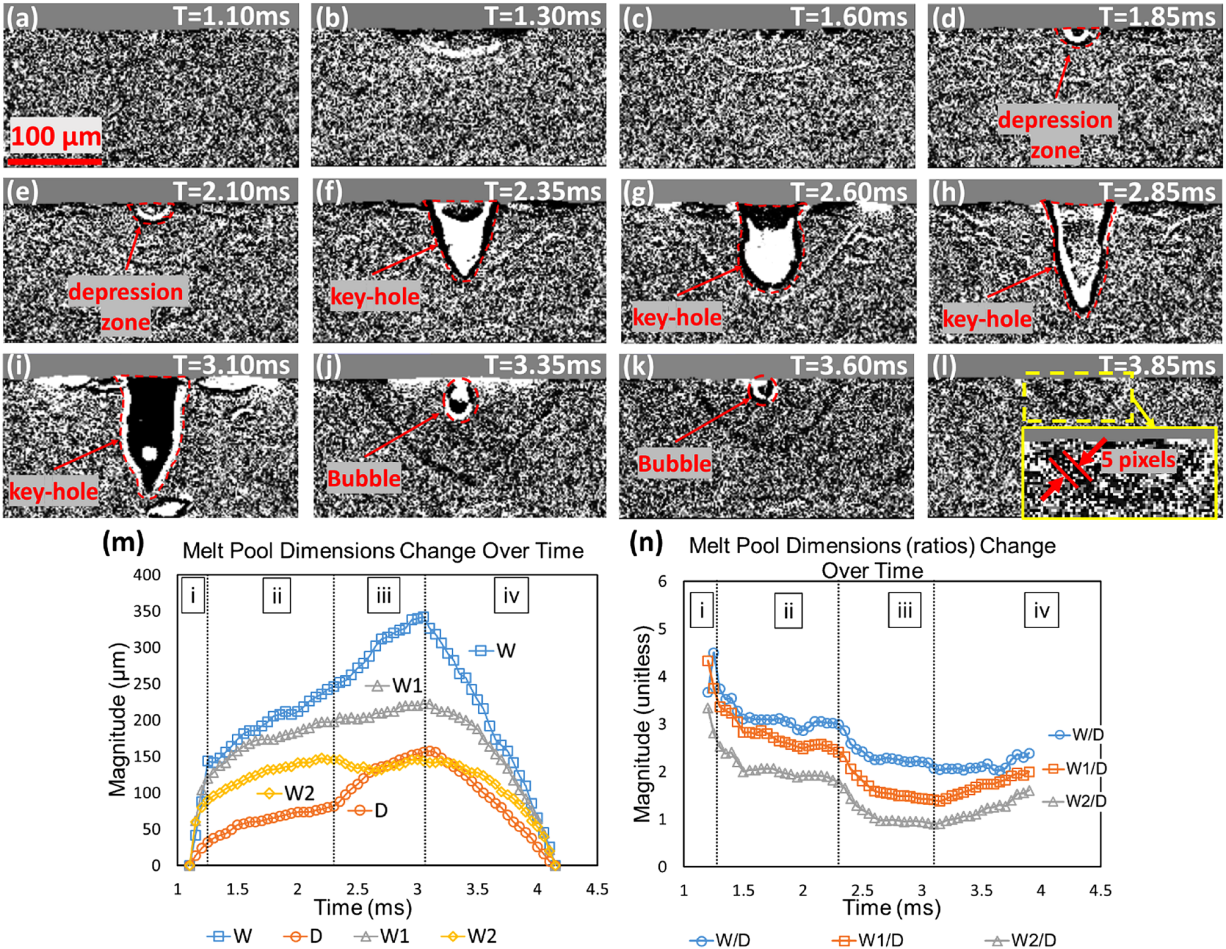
(a–l) The selected high-speed X-ray images of the melt pool of Trial #20. From the X-ray observations, phenomena such as keyholes and bubbles can be seen. (m) The melt pool dimensions change over time where blue squares, orange circles, gray triangles, and yellow diamonds represent the size of *W*, *D*, *W*_1_, and *W*_2_, respectively. Intervals i, ii, iii, and iv represent initial heating, conduction melting, keyhole melting, and cooling, respectively. (n) The melt pool dimension ratios change over time where blue circles, orange squares, and gray triangles represent the *W*/*D*, *W*_1_/*D*, and *W*_2_/*D*, respectively. Notice that *W* and *D* have a constant rate of change during the cooling process. The uncertainties of the extracted dimension values are ± 4 µm.
